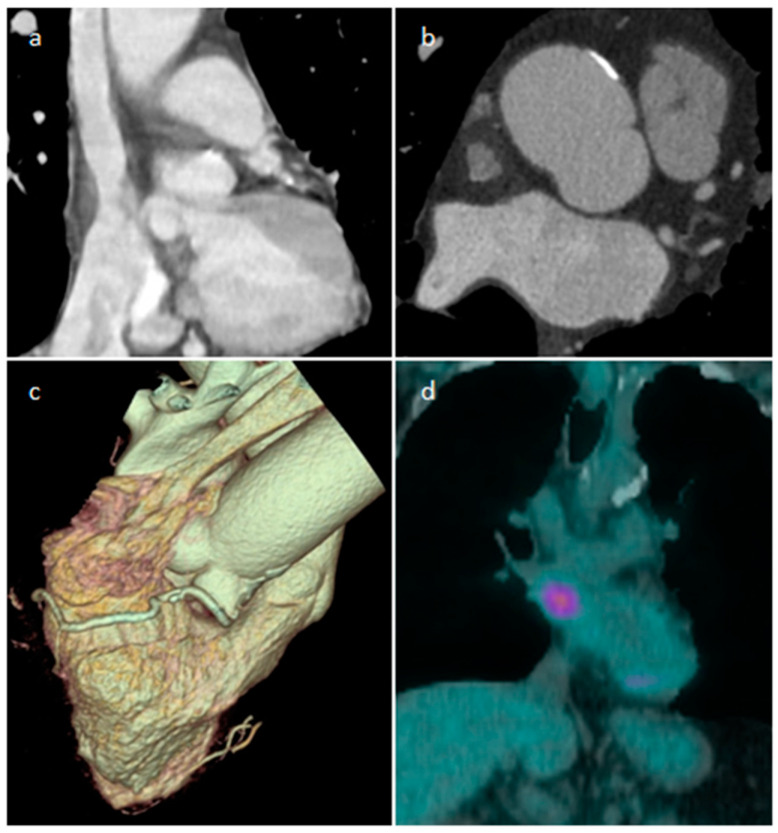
A 65-year-old male patient with lipomatous hypertrophy of the interatrial septum on coronal and axial CT images (a,b), volume rendering (c), which showed focal uptake of FDG in PET/CT scan (d).
